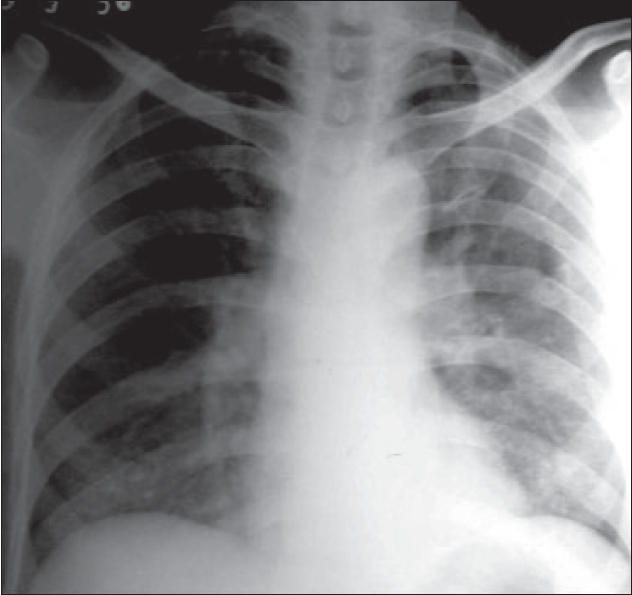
Chest radiograph (postero-anterior view) of the patient without any gross abnormality
